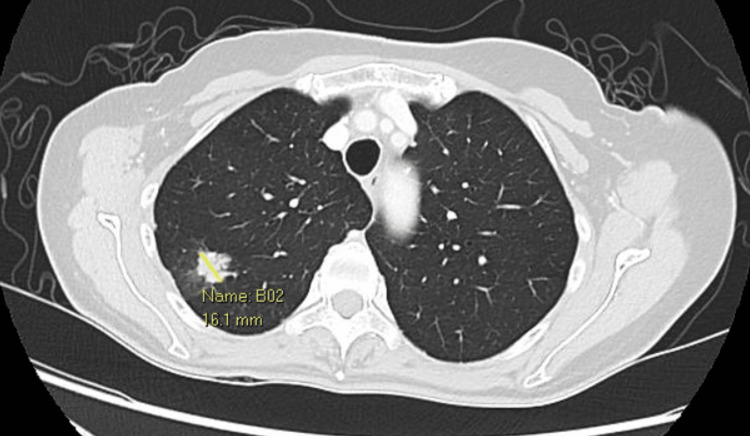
Axial cut of the chest CT showing a 1.6 cm solid nodule in the upper lobe of the right lung with perilesional ground glass changes, indeterminate for disease extension versus perilesional hemorrhagic change. This was consistent with biopsy-proven pulmonary metastasis secondary to uterine leiomyosarcoma. CT: computed tomography
